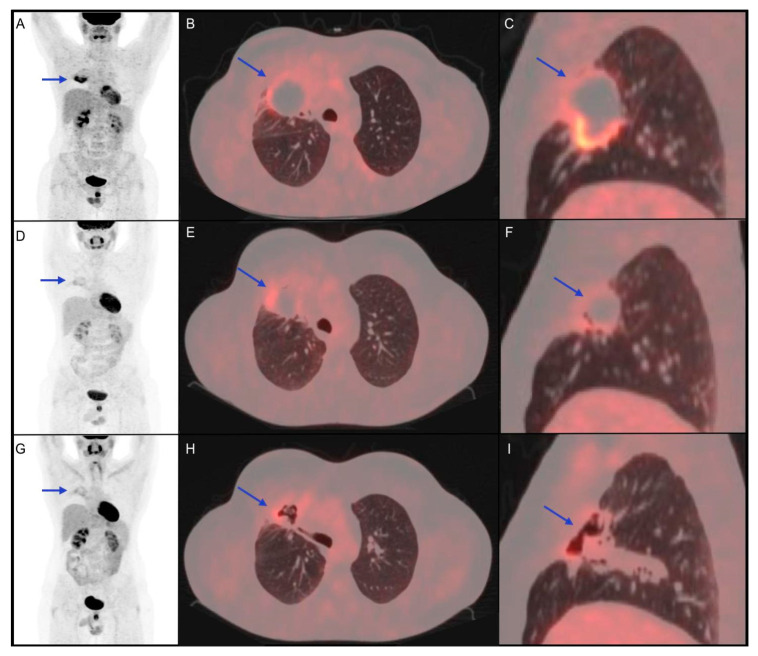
Clinical case of a 34-year-old male patient affected by diffuse large B-cell lymphoma diagnosed with biopsy of mediastinal mass, with right lung involvement, in June 2016. He underwent radio-chemotherapy with R-CHOP, R-DHAP and R-IEV with a subsequent stem cell transplant in June 2017. In March 2018, the persistence of the right lung lesion led haematologists to choose a following therapeutic option immune checkpoint inhibitor (pembrolizumab). (A–C) 18F-FDG PET/CT performed before the start of immunotherapy shows a metabolically active right lung lesion (blue arrow, SUVmax 8.7). (D–F) 18F-FDG PET/CT performed 5 months after the start of immuno-treatment shows partial metabolic response of the known lesion with a reduction of SUVmax (blue arrow, SUVmax 4.2). (G–I) 18F-FDG PET/CT 4 months after the end of immunotherapy shows further metabolic reduction (SUVmax 3.5) of the lung lesion, which appeared excavated on CT coregistered to PET images. The patient was followed-up with stable disease until December 2020, when progression disease was documented.
